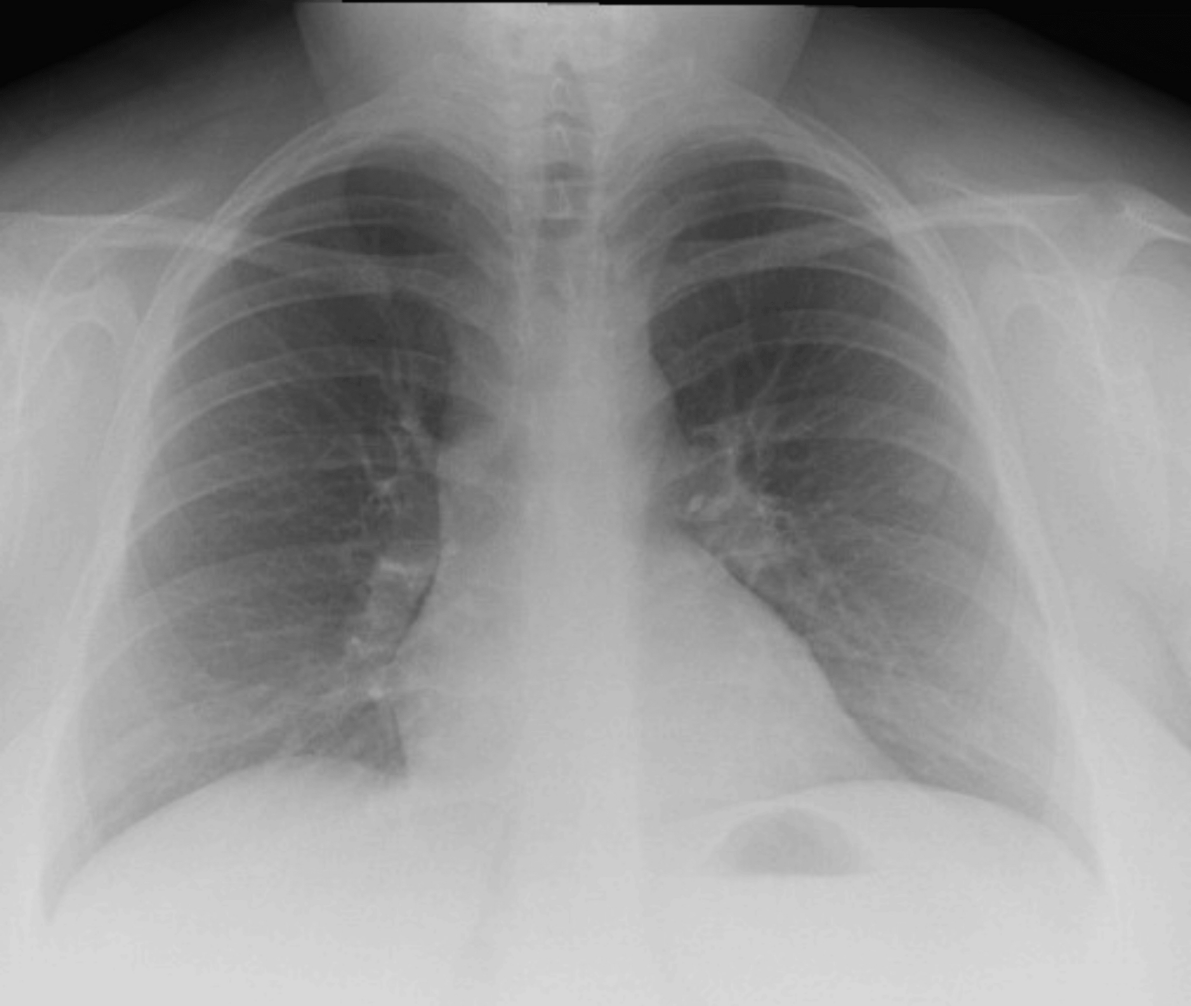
Follow-up X-ray after treatment shows complete resolution of reticular interstitial shadowing.
